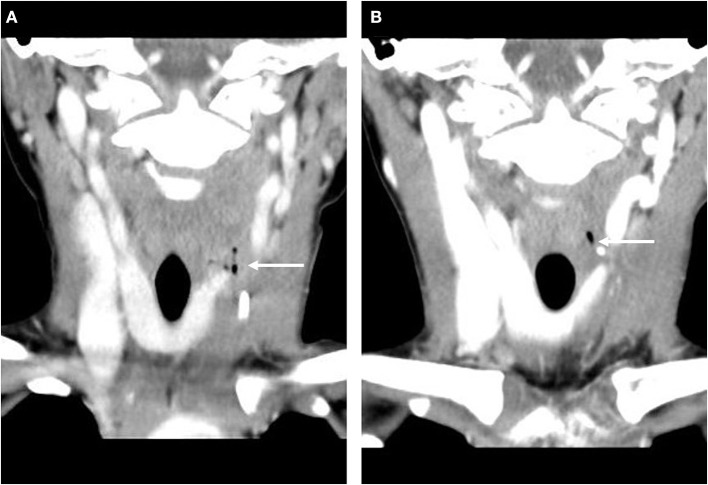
CT coronal scan showed air density before primary operation (A, arrow) and reoperation (B, arrow).
